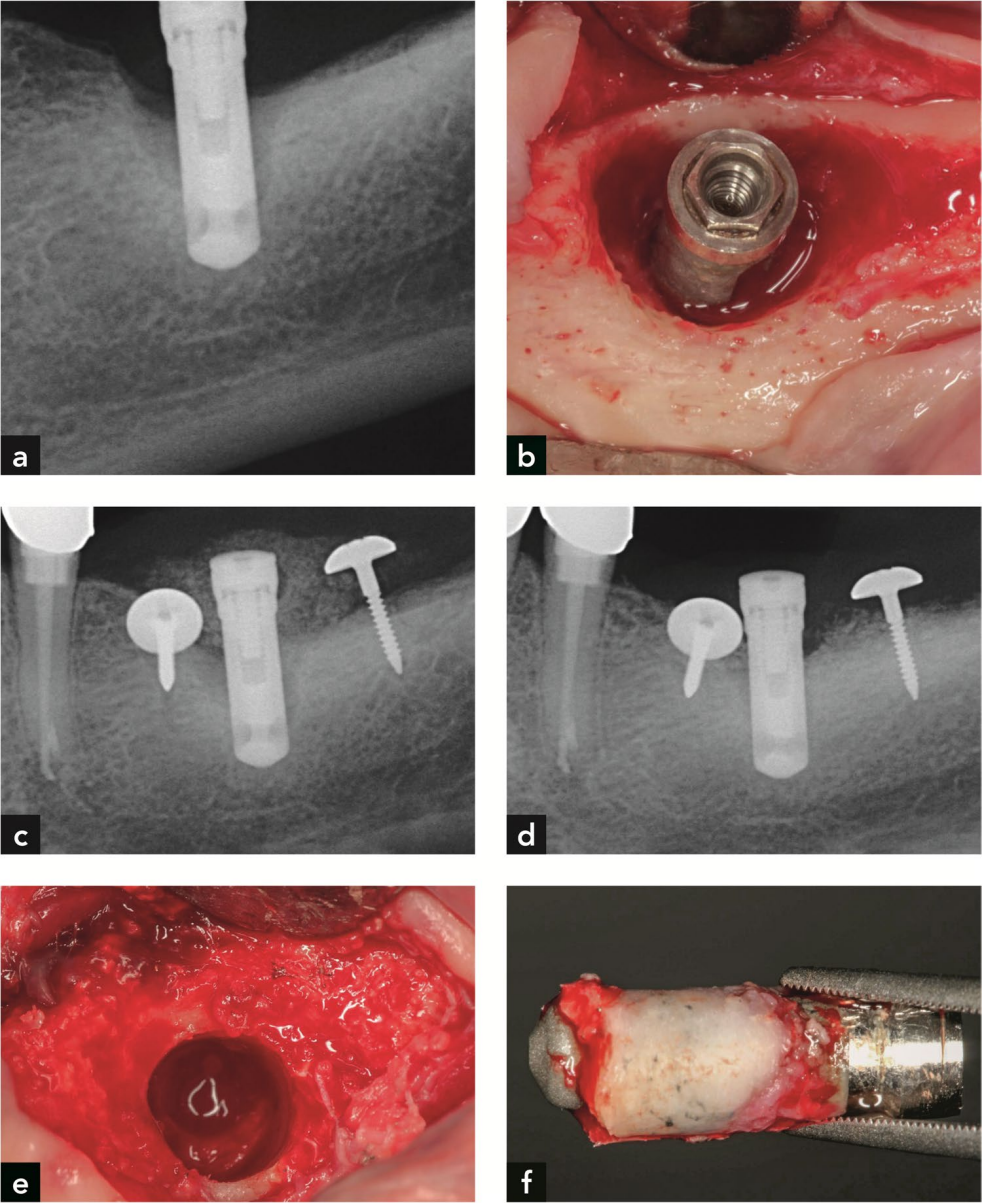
Implant #1. (a) Radiographs demonstrating peri-implant bone loss on the day of electrolytic treatment. (b) Implant and bone after debridement and electrolytic cleaning. (c) Radiograph after GBR with the use of umbrella screws to tent up the non-contained bone defect. (d) Radiograph on the day of explantation. (e) Resident bone after implant retrieval with a trephine bur, and (f) Retrieved implant with surrounding bone
